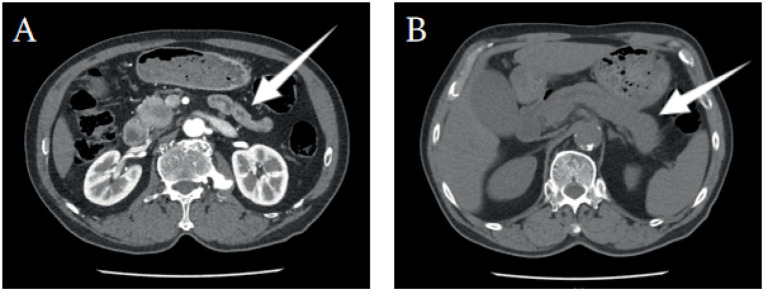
Upper Abdominal Enhanced CT Images. (A) Arterial phase CT scan showing enlargement of the uncinate process of the pancreas with uneven density, presenting patchy low-density areas. The common bile duct and main pancreatic duct are obstructed, and the adjacent bile duct and duodenal wall are thickened. The contrast-enhanced scan shows mild, uneven enhancement. (B) Venous phase CT scan showing a mass-like lesion in the uncinate process of the pancreas, with pancreatic parenchymal enlargement and dilatation of the bile ducts.
